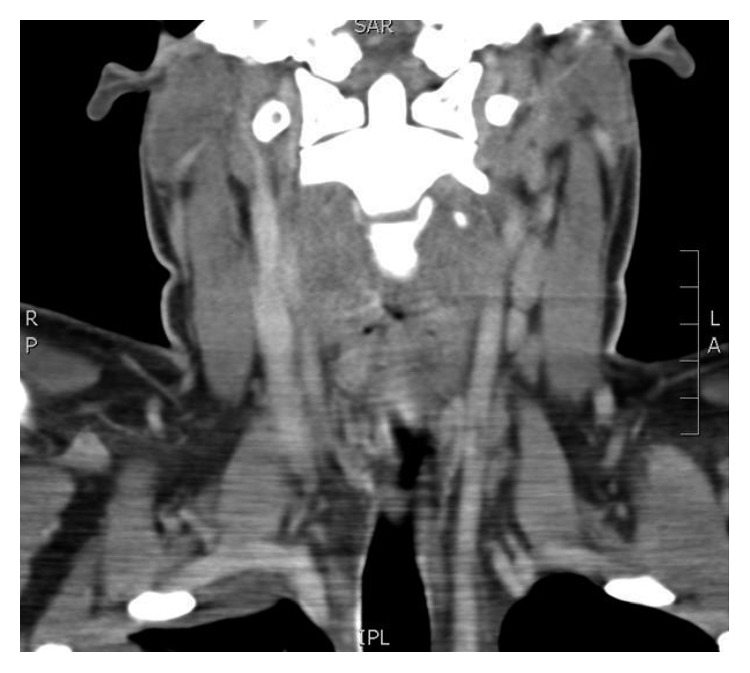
CT neck. No venous thrombosis.
